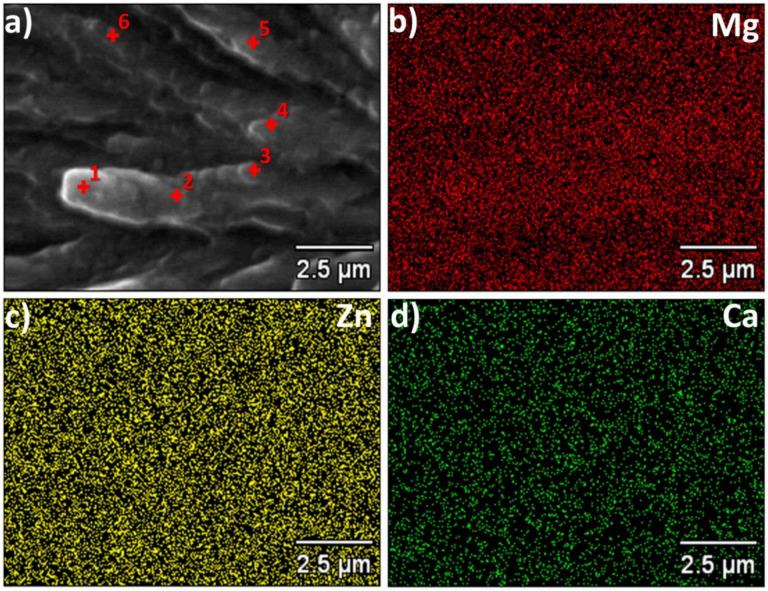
The X-ray microanalysis of the ribbon cross section with elemental distribution. For the marked points compositions are provided in Table 1. (a) SEM image with marked location of point analysis, (b) magnesium distribution, (c) zinc distribution and (d) calcium distribution.
